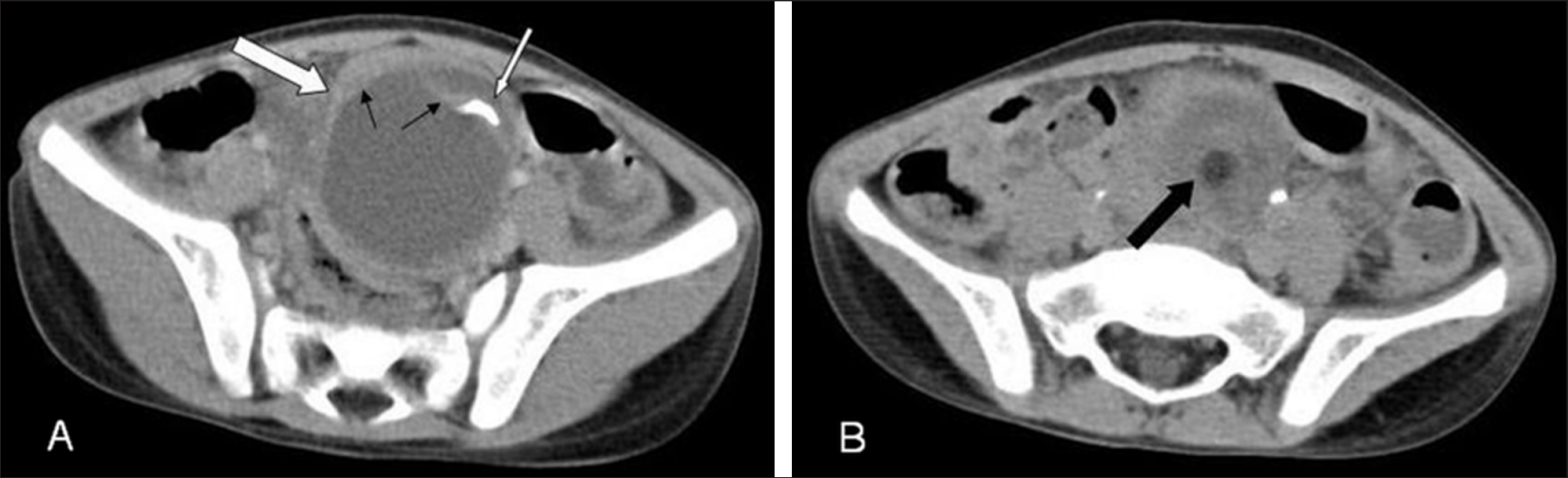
Non-contrast (A) and contrast enhanced (B) computed tomography (CT) scans show a calcified (white thin arrow), encompasses an area of fat density (thick black arrow) and septa (thin black arrow) 6 cm-sized smooth-walled cystic mass (white thick arrow) distorting the surrounding structures, consistent with teratoma of a 5-year-old girl in the pelvic region. The post-operative diagnosis of the case was right ovarian teratoma and ovarian torsion.
